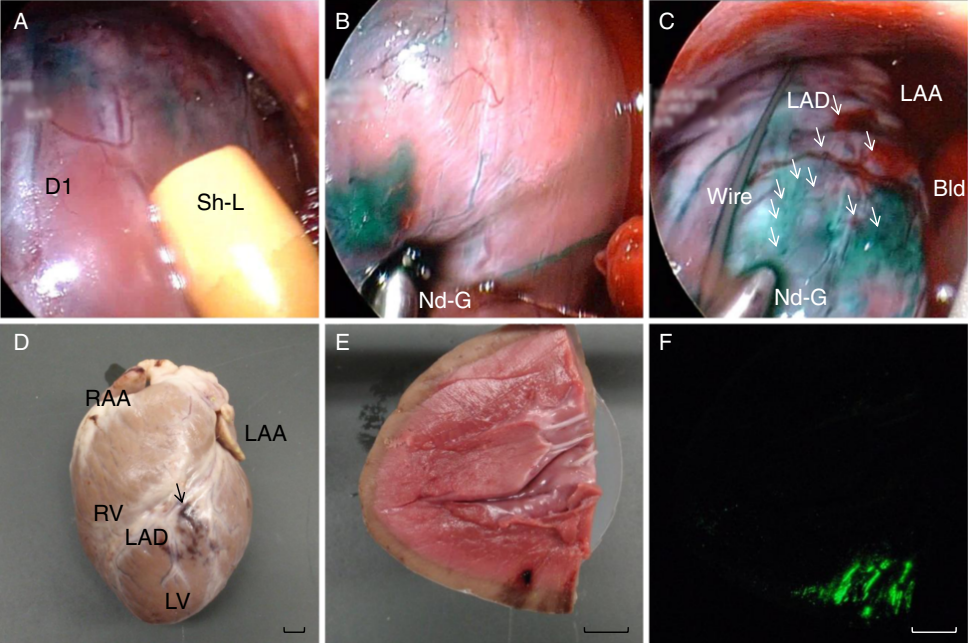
Demonstration of cell transplantation with needle with grip (Nd-G). A mixture of silicon beads, indocyanide green, ultrasonographic and fluoroscopic contrast medium was injected into the myocardium by Nd-G. A: Two sheaths were inserted into the pericardial space, Sh-S with R-70 and Sh-L with Nd-G. Coronary vessels such as the first diagonal branch (D1) were clearly visualized to avoid injuries. B: Nd-G was inserted through Sh-L and placed at the puncture site while avoiding coronary vessels. C: Injections were performed 20 times (white arrows). Intraventricular injections due to strong motion artifacts caused a slight hemorrhage (Bld) that spontaneously stopped without hemodynamic change. Macroscopic evaluation (D), a cross section of injected sites (E), and a cross section with fluorescence filter (F) showed that the transmural injection was successfully performed in the anterolateral wall of the left ventricle. Bld: blood; LAA: left atrial appendage; LAD: left anterior descending artery; LV: left ventricle; RAA: right atrial appendage; RV: right ventricle; Wire: a guide wire; Sh-L, Sh-S, Nd-G; as described in Figure 1.
